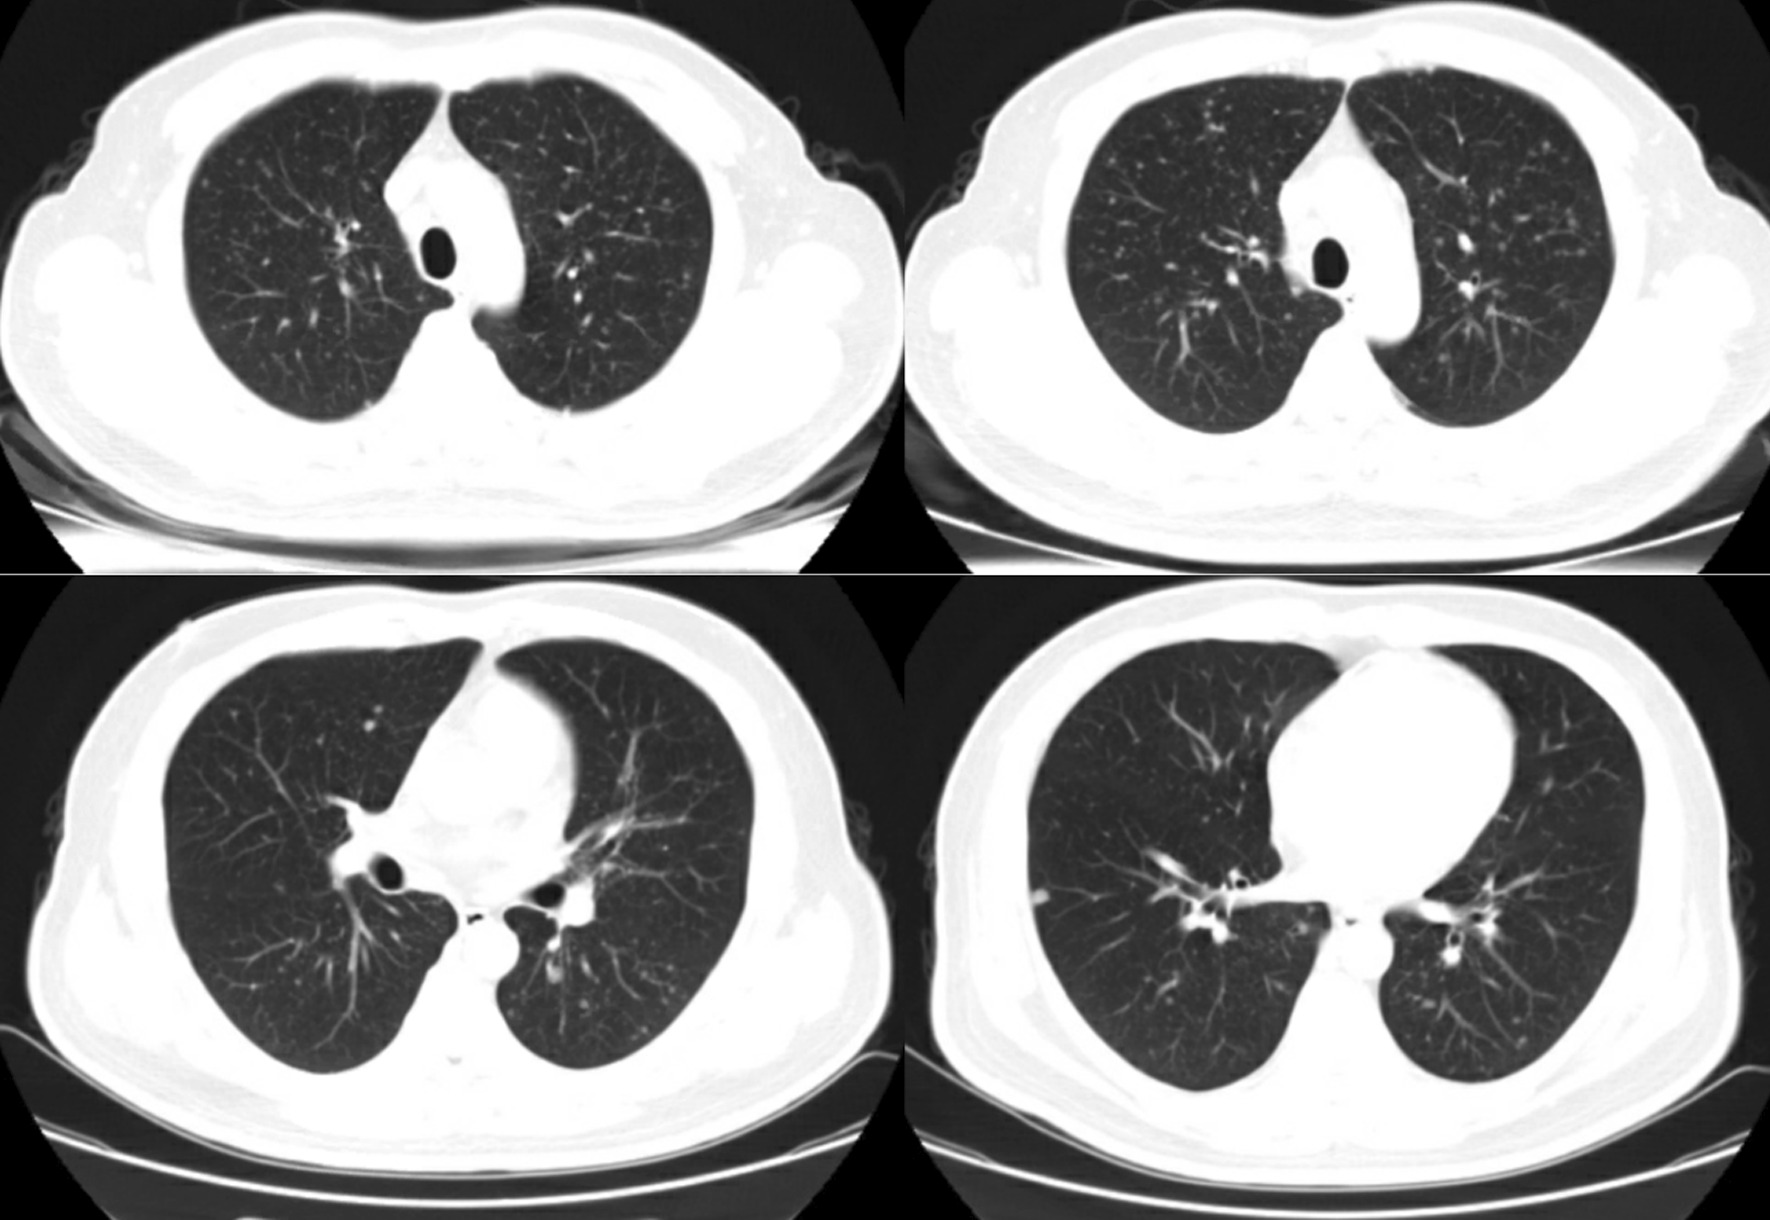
Presentation of chest CT scan. Chest scan showed diffuse small, well-defined nodules of different sizes in both lungs
